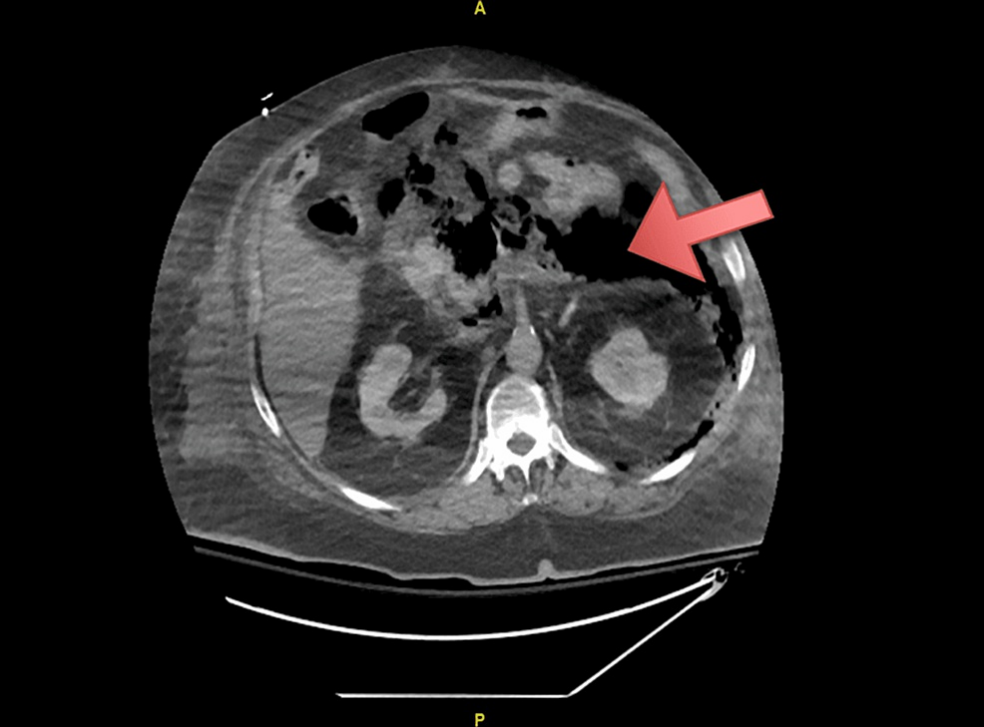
CT of the abdomen and pelvis revealing extensive gas collection in the retroperitoneum extending to the root of the mesentery and left pararenal space consistent with emphysematous pancreatitis.
